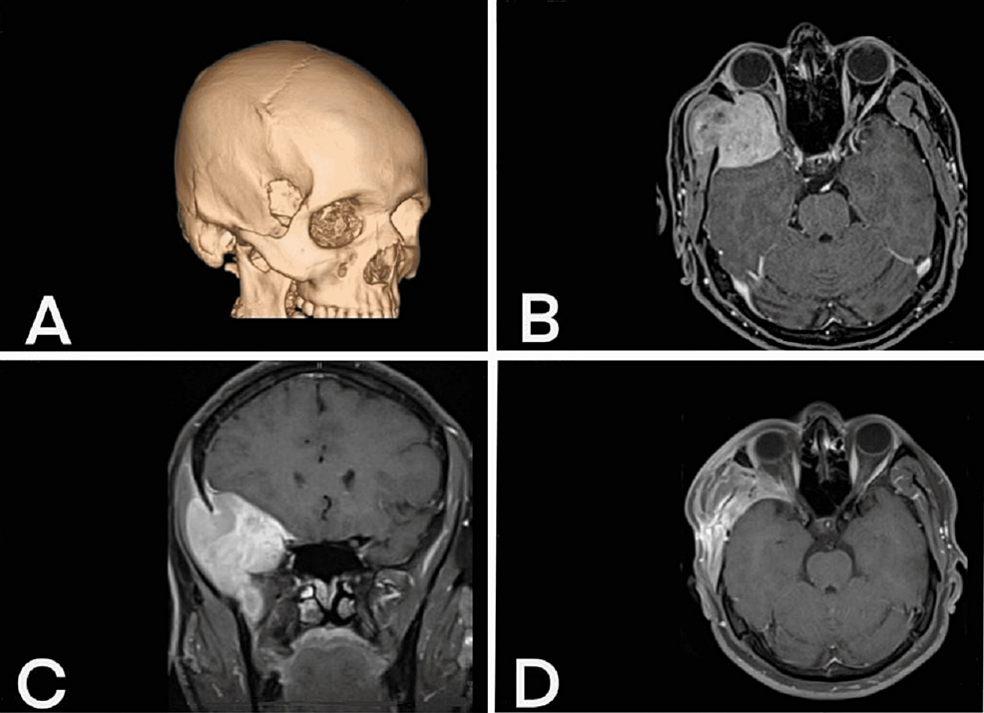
(A) Three-dimensional reconstructed CT scan image showing the destruction of the adjacent sphenoid bone at the superolateral orbit. (B) Axial gadolinium-enhanced T1-weighted preoperative MRI demonstrating lobulated extra-axial heterogeneous enhancing mass at the right frontotemporal region composed of extracranial and intracranial components causing right orbital nerve compression. (C) Coronal gadolinium-enhanced T1-weighted preoperative MRI showing lobulated extra-axial enhancing mass at the right frontotemporal region composed of extracranial and intracranial components. (D) Axial gadolinium-enhanced T1-weighted postoperative MRI showing soft tissue swelling and edema at the superolateral orbit and temporal fossa.
